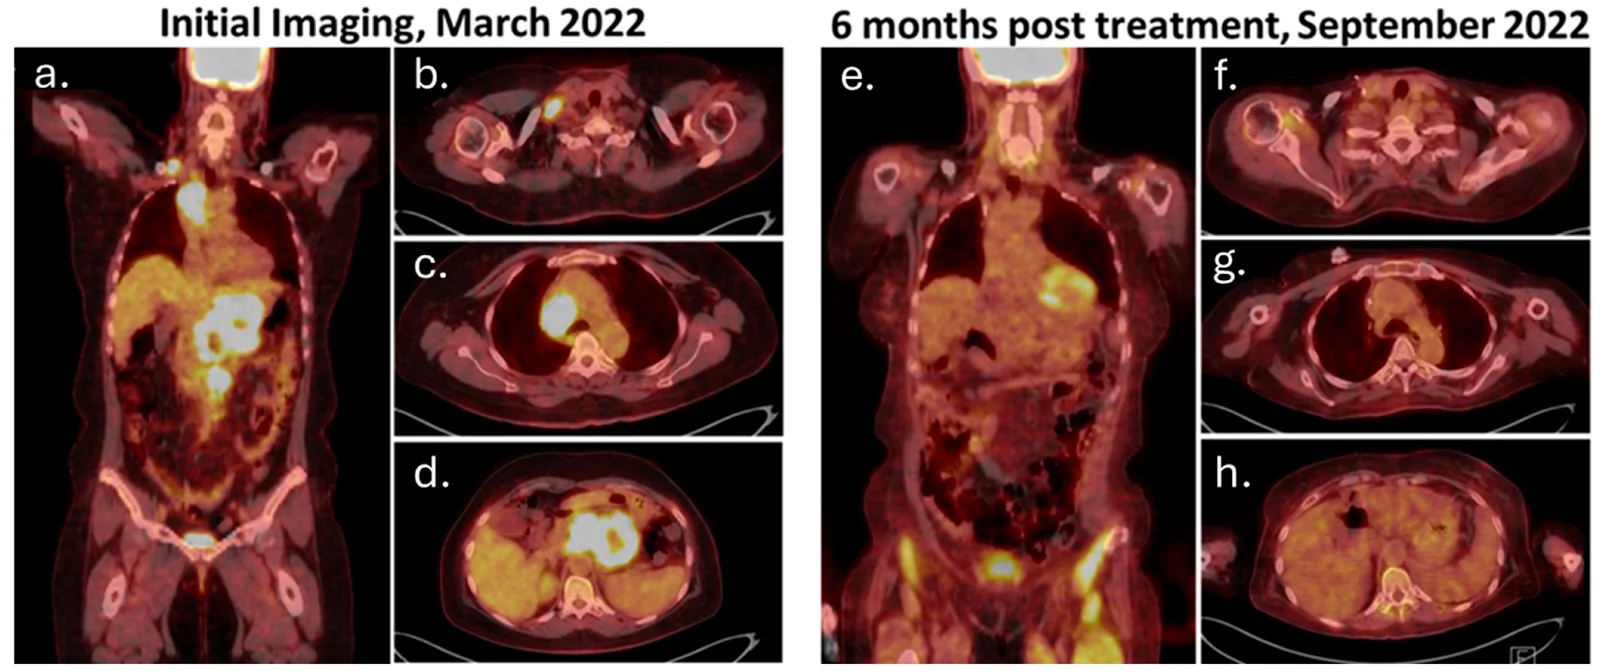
Baseline and post-treatment PET imaging demonstrating complete metabolic response. Initial PET imaging obtained at diagnosis in March 2022 demonstrated FDG-avid primary gastric disease with extensive nodal involvement. (a) shows the baseline coronal PET image demonstrating the primary gastric mass with conglomerate gastrohepatic and celiac axis lymphadenopathy measuring 76 mm, as well as FDG-avid adenopathy involving the right neck, right paratracheal, and para-aortic regions. (b) shows a right supraclavicular lymph node measuring 11 mm with SUV 7.9. (c) shows a right paratracheal lymph node measuring 42 mm with SUV 14.3. (d) shows the primary gastric mass with conglomerate gastrohepatic and celiac adenopathy measuring 76 mm with SUV 14.8. Follow-up PET imaging after nivolumab-based therapy is shown in (e–h) and demonstrates complete metabolic response, with resolution of FDG avidity in the right supraclavicular lymph node, right paratracheal lymph node, and primary gastric mass, along with complete anatomic resolution of the gastric mass. FDG, fluorodeoxyglucose; PET, positron emission tomography; SUV, standardized uptake value.
